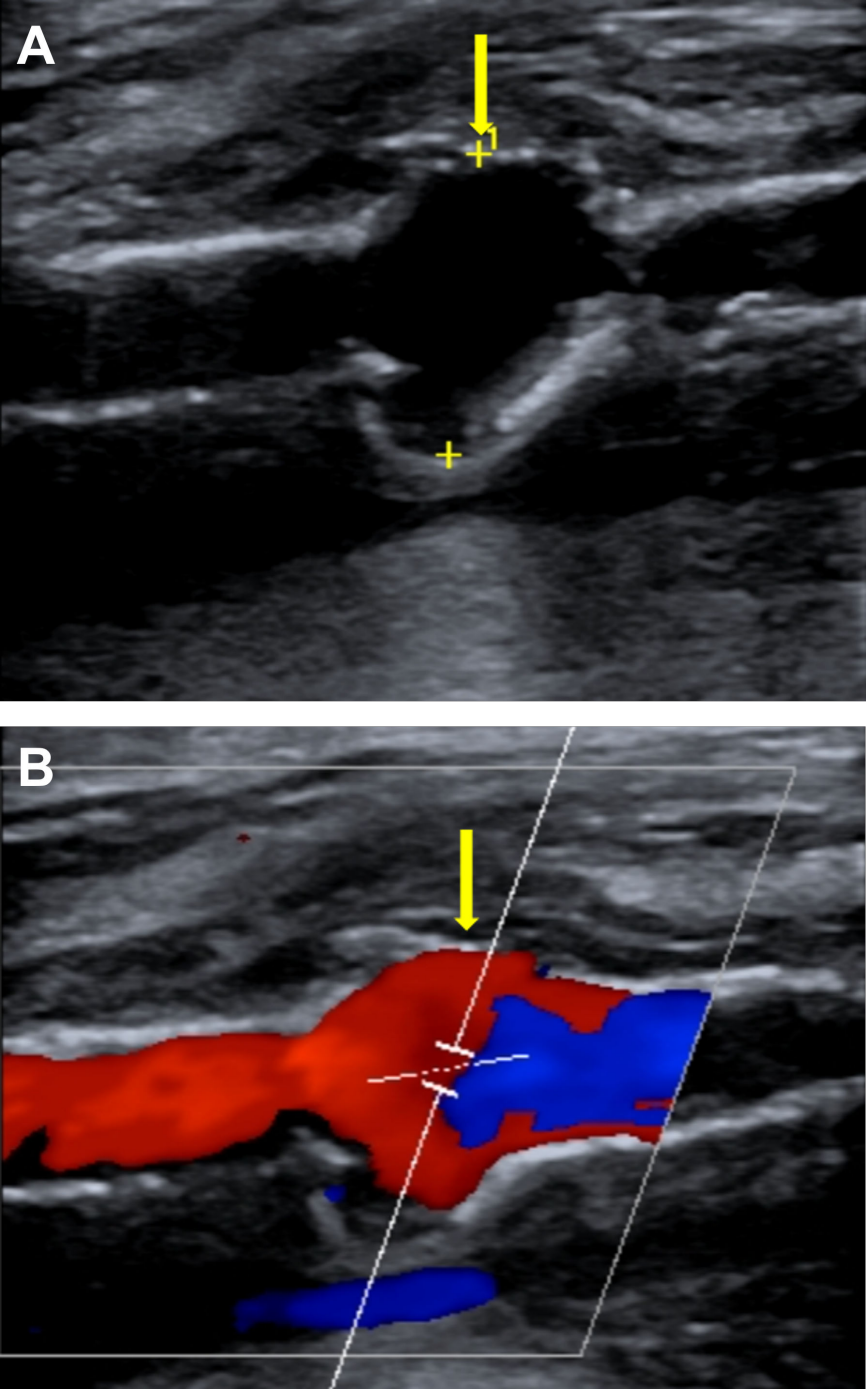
Ultrasound of the right superficial femoral artery. The images show representative longitudinal views of the vessel (A) without Doppler and (B) with Doppler. Yellow arrows indicate the pseudoaneurysm.
